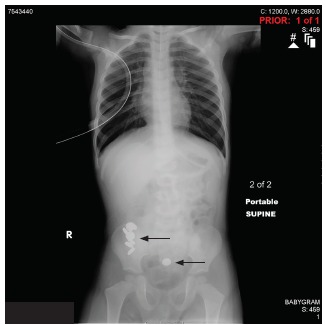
Supine radiograph showing progression of multiple button batteries (arrows) after 14 hours of whole-bowel irrigation.
